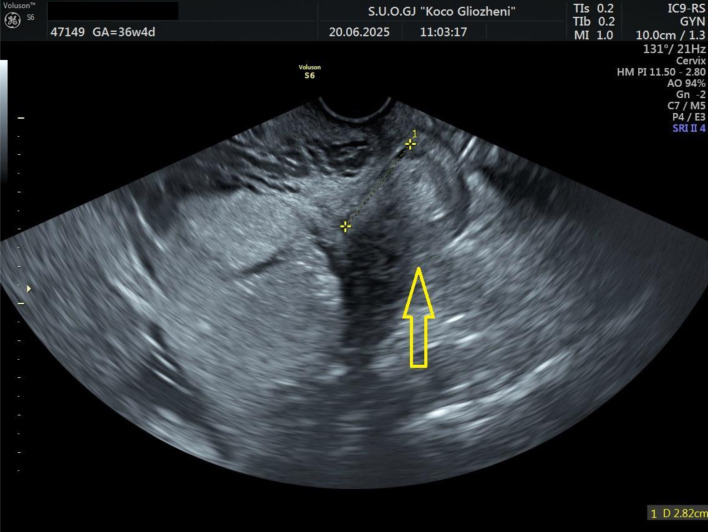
Ultrasound examination showing placental tissue invading the lower myometrium (arrow).
